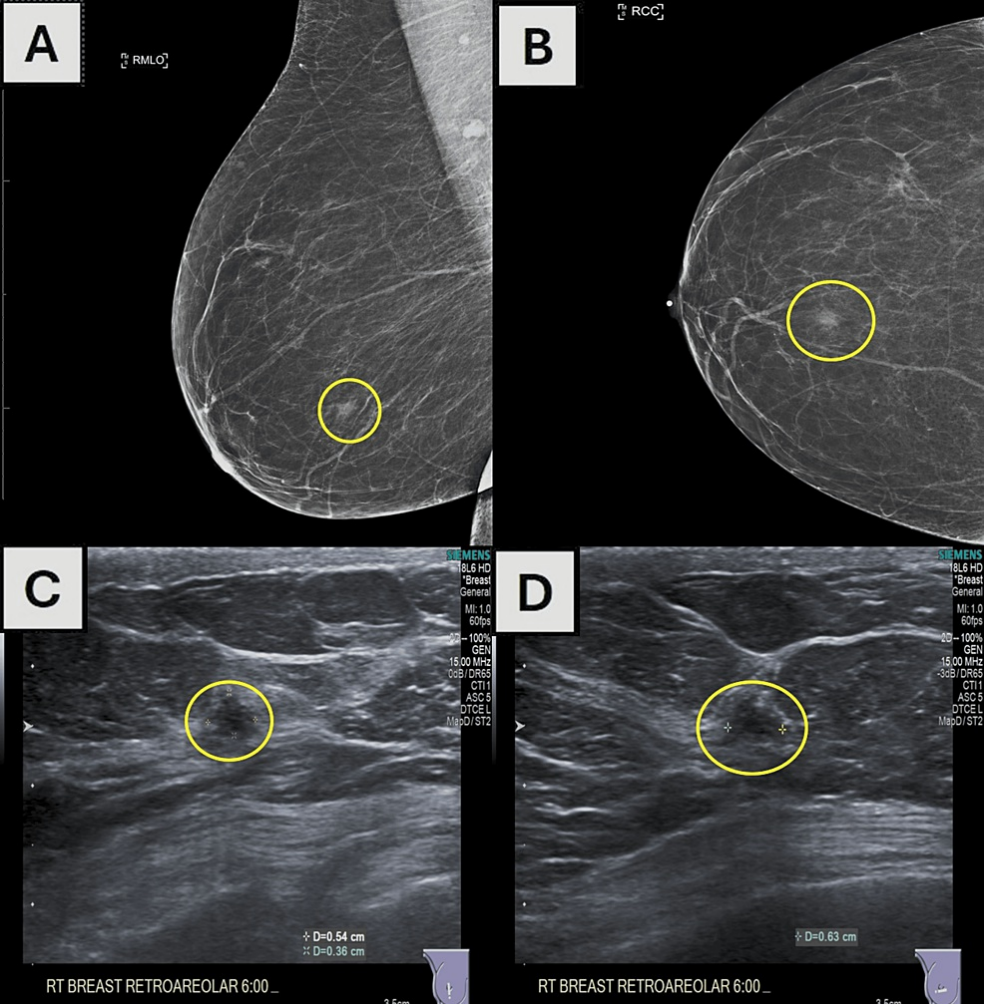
Comprehensive screening and diagnostic imaging of right breast lesion. Initial mammographic images and follow-up ultrasound imaging of the right breast: (A) right mediolateral oblique (RMLO) view and (B) right craniocaudal (RCC) view from screening mammography revealing focal asymmetry in the retroareolar plane, as outlined by the white ovals; (C) ultrasound image depicting a hypoechoic, irregularly shaped mass measuring 0.5 cm × 0.4 cm × 0.6 cm at the retroareolar 6:00 position, as outlined by the white ovals; (D) ultrasound image without internal Doppler color flow corresponding to the area of suspicion. No skin thickening, retraction, or abnormal lymph nodes were observed. The constellation of these findings, not previously noted, was assigned a BI-RADS 5 assessment, suggestive of a high probability of malignancy.
